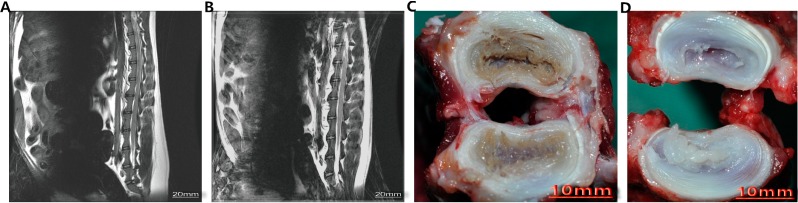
The T2-weighted magnetic resonance imaging (MRI) and morphology of the beagle model of Intervertebral Disk Degeneration (IDD). (A) The MRI of the lumbar disc after disc puncture; (B) The MRI of the lumbar disc without disc puncture; (C) The morphology of the lumbar disc after disc puncture; (D) The morphology of the normal lumbar disc.
